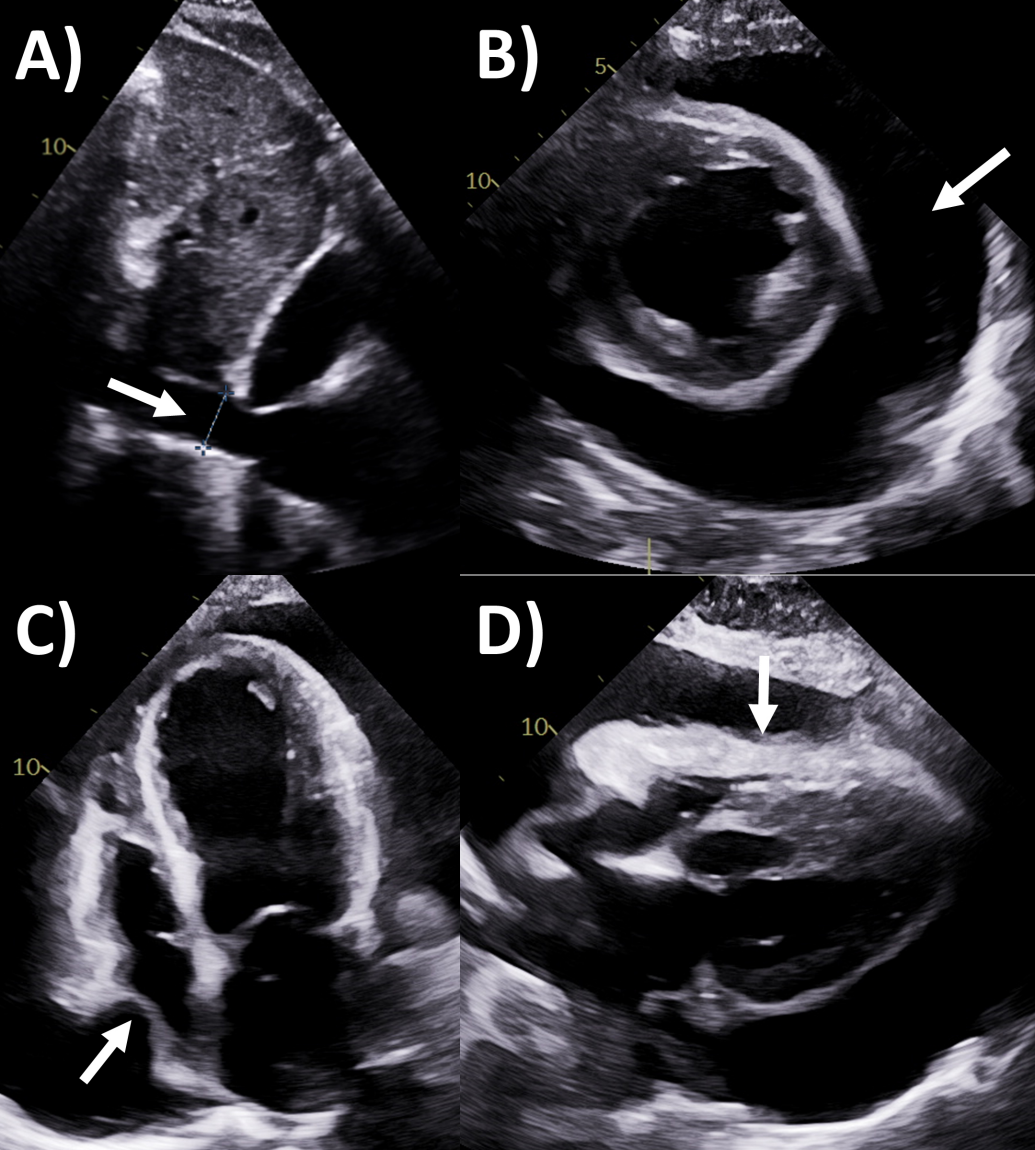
Echocardiography evaluation of pericardial tamponade. (A)
